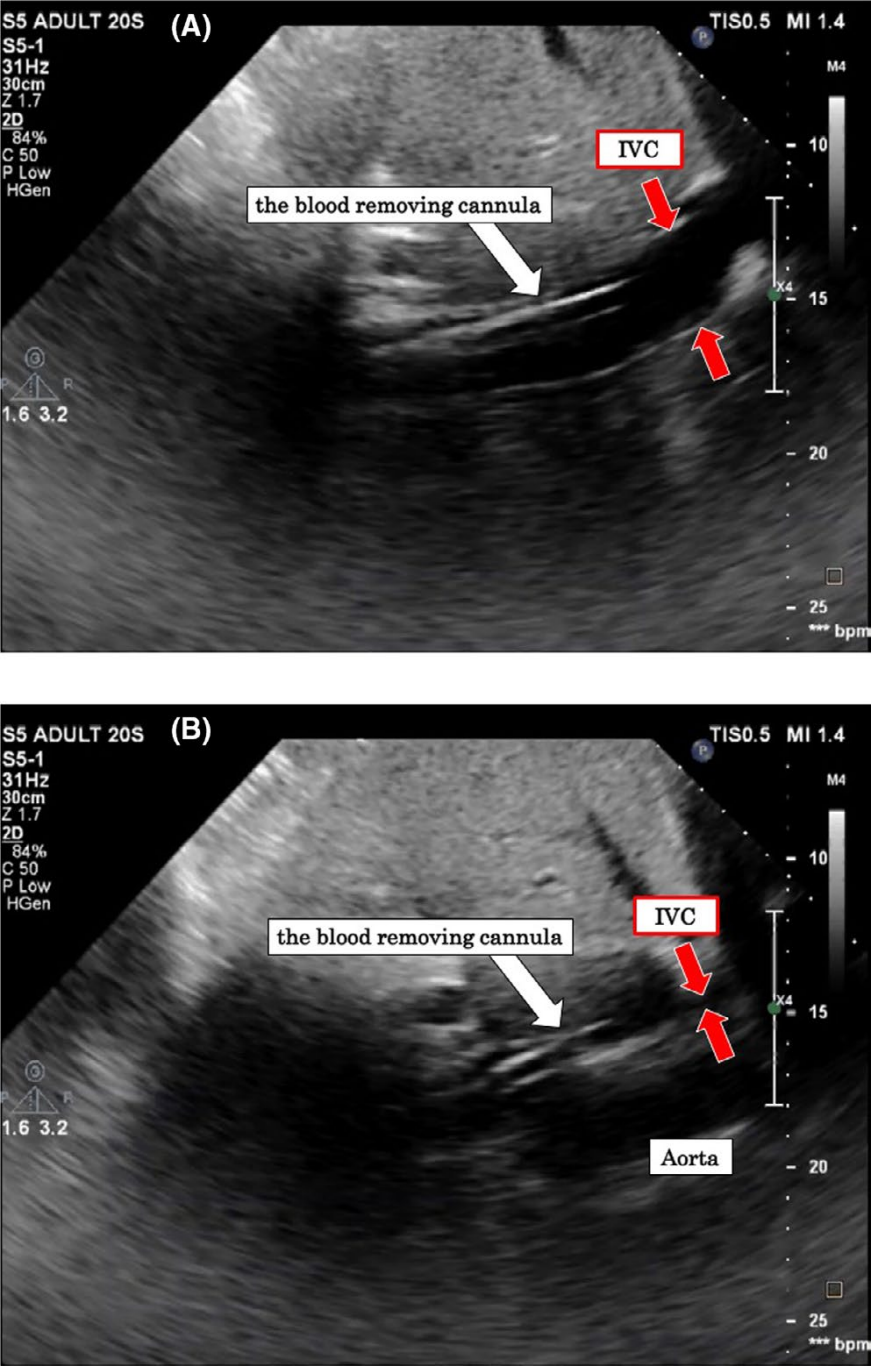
Ultrasonographic findings during reproduction of the episode. The diameter of the inferior vena cava was maintained, and no collapse was observed under baseline conditions (A). The inferior vena cava collapsed following straining (B). At the time of straining, the drainage of blood via the blood‐drainage cannula was interrupted, and a drop in blood flow of extracorporeal membrane oxygenation was induced
